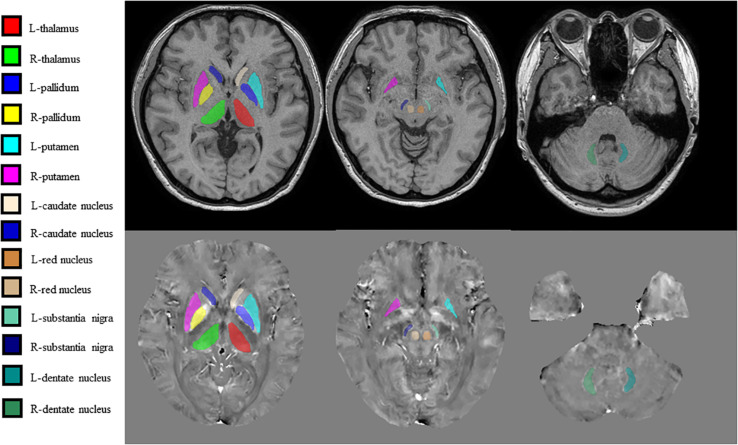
Regions of interest (ROIs) were traced directly on the quantitative susceptibility mapping (QSM) image and T1-weighted (T1W) images to remove veins with high positive susceptibility values on QSM.
